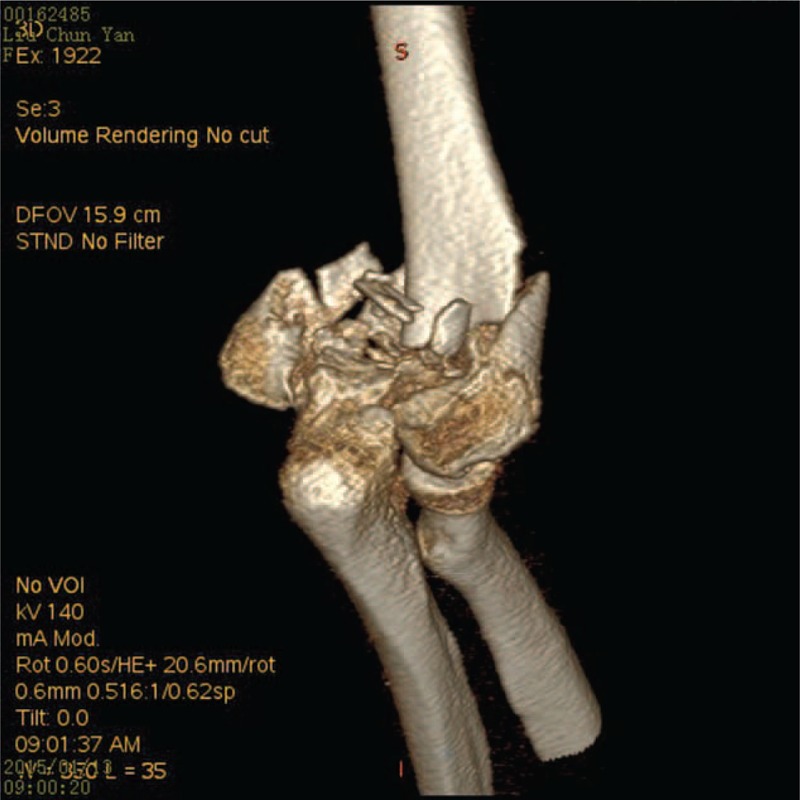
3D reconstructed computed tomography images of a 53-year old woman with a comminuted intercondylar humeral fracture of the right arm.
